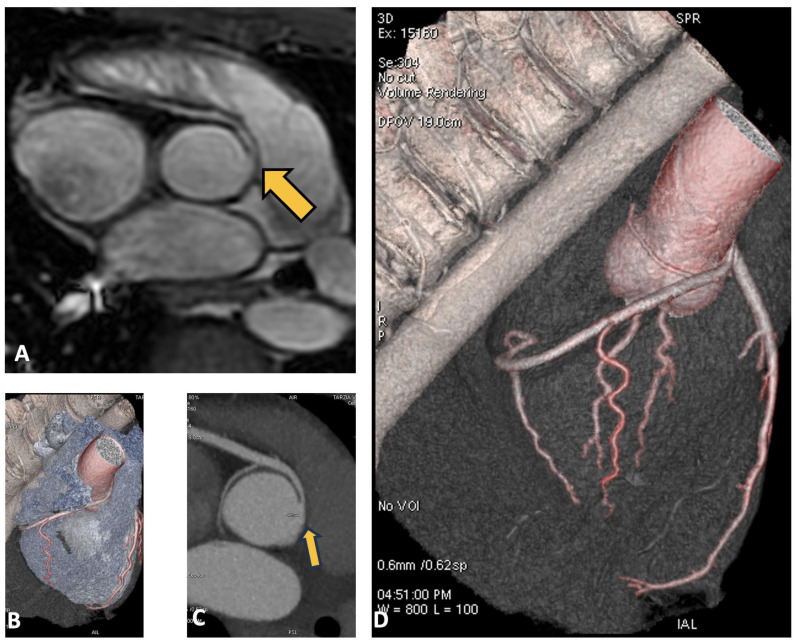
In panel (A), the cardiac MRI in the coronary study sequence shows the right coronary artery, indicated by the yellow arrow, originating from the left Valsalva sinus. In panels (B–D), similar images from the CCTA are shown, with the right coronary artery originating from the left sinus.
